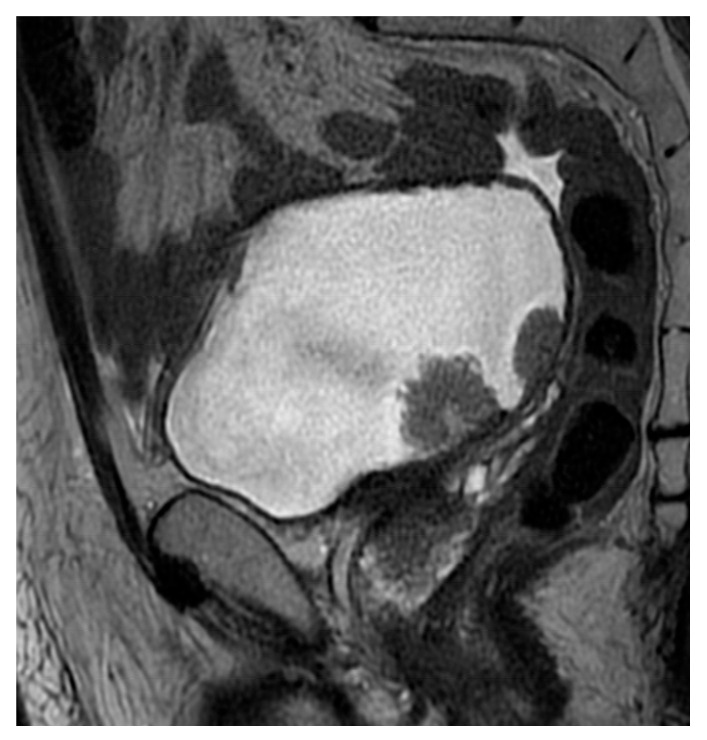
T2w sagittal Multiparametric MRI in a 70-year-old patient demonstrates two synchronous bladder lesions with distinct morphologic and functional characteristics.
